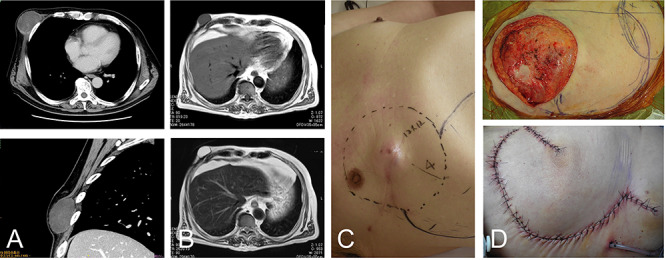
76-year-old male with recurrent myxofibrosarcoma. Computed tomography revealed the initial 6-cm subcutaneous lesion of the patient at 73 years of age (A); 3 years after resection of the initial lesion, T1- and T2-weighted images show a 3-cm recurrent lesion with high-signal intensities (B-upper) and (B-lower), respectively. Photograph showing protuberant subcutaneous lesion (C); defect after resection of the tumor with the surrounding tissue (D-upper). The defect was reconstructed with a thoracoabdominal flap (D-lower).
